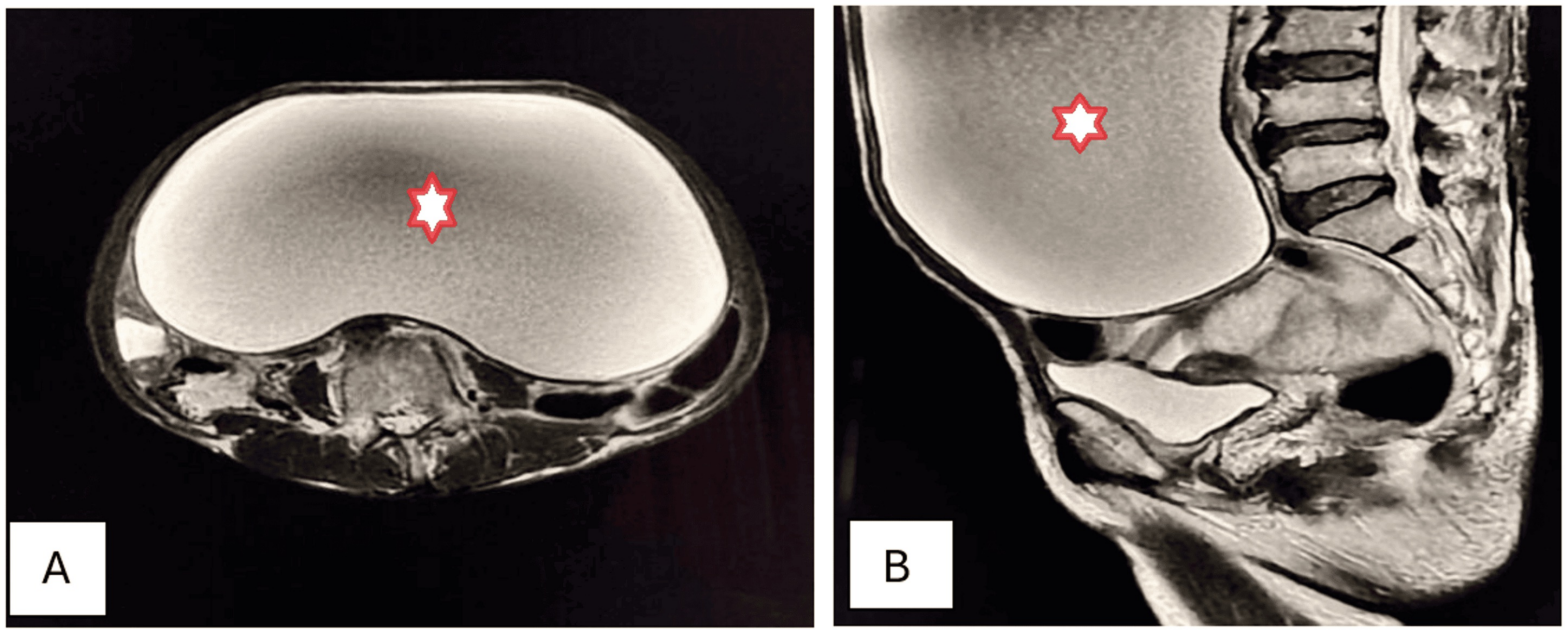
Serous cystadenoma of the right ovary (stars). Axial (A) and sagittal (B) T2W MRI shows a huge, well-circumscribed, unilocular, abdominopelvic, cystic mass arising from the pelvis and extending up to the upper abdomen with mass effect and peripheral displacement of the bowel loops, which is consistent with serous cystadenoma of the ovary. T2W: T2-weighted
